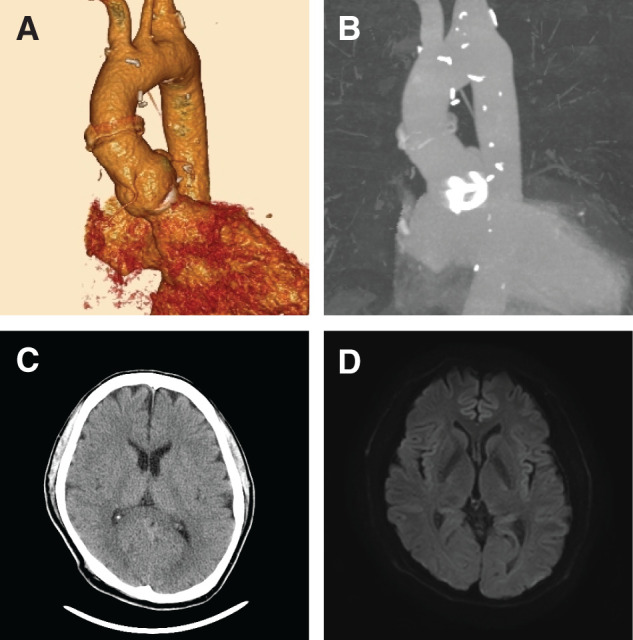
Postoperative imaging findings. (A, B) Cardiac CT following the Bentall procedure (A) and MIP images (B). (C) Head CT showing no hemorrhagic lesions. (D) Postoperative MRI diffusion-weighted images showing no evidence of infarction. MIP, maximum intensity projection
